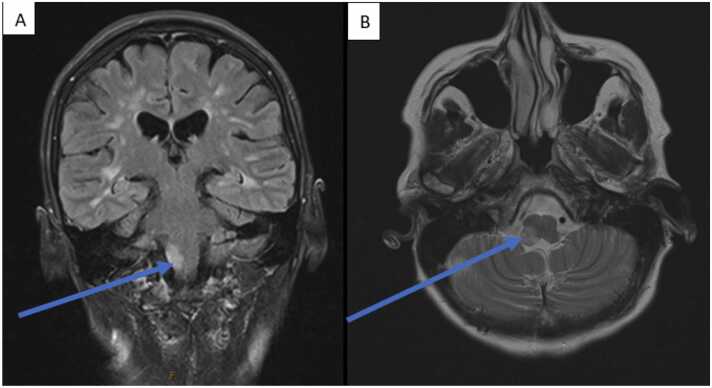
Repeat MRI showing stable appearances of the supratentorial demyelinating lesions, persisting but improved enhancement in the right basal ganglia and bilateral white matter associated with known cryptococcosis, and a new signal hyperintensity with leptomeningeal enhancement in the right medulla with an enhancement pattern atypical for demyelination. A = T2 FLAIR, coronal view post gadolinium administration; B = T2, transverse view.
